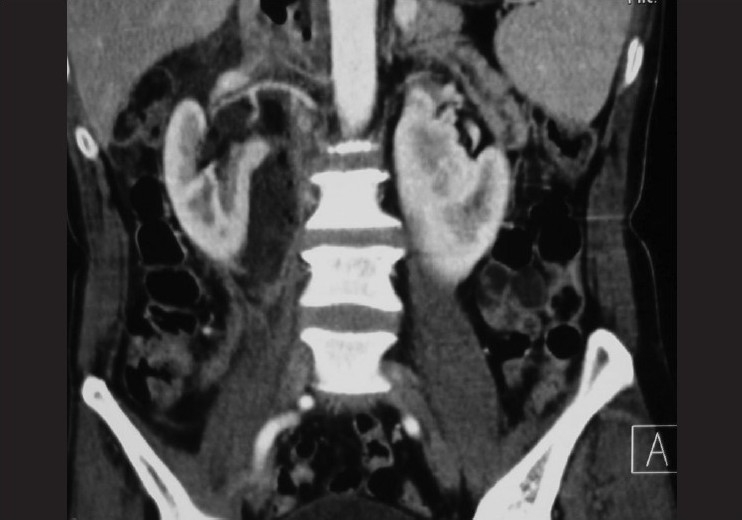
Coronal thick MPR CT image showing right perinephric abscess and inflammed appendix
